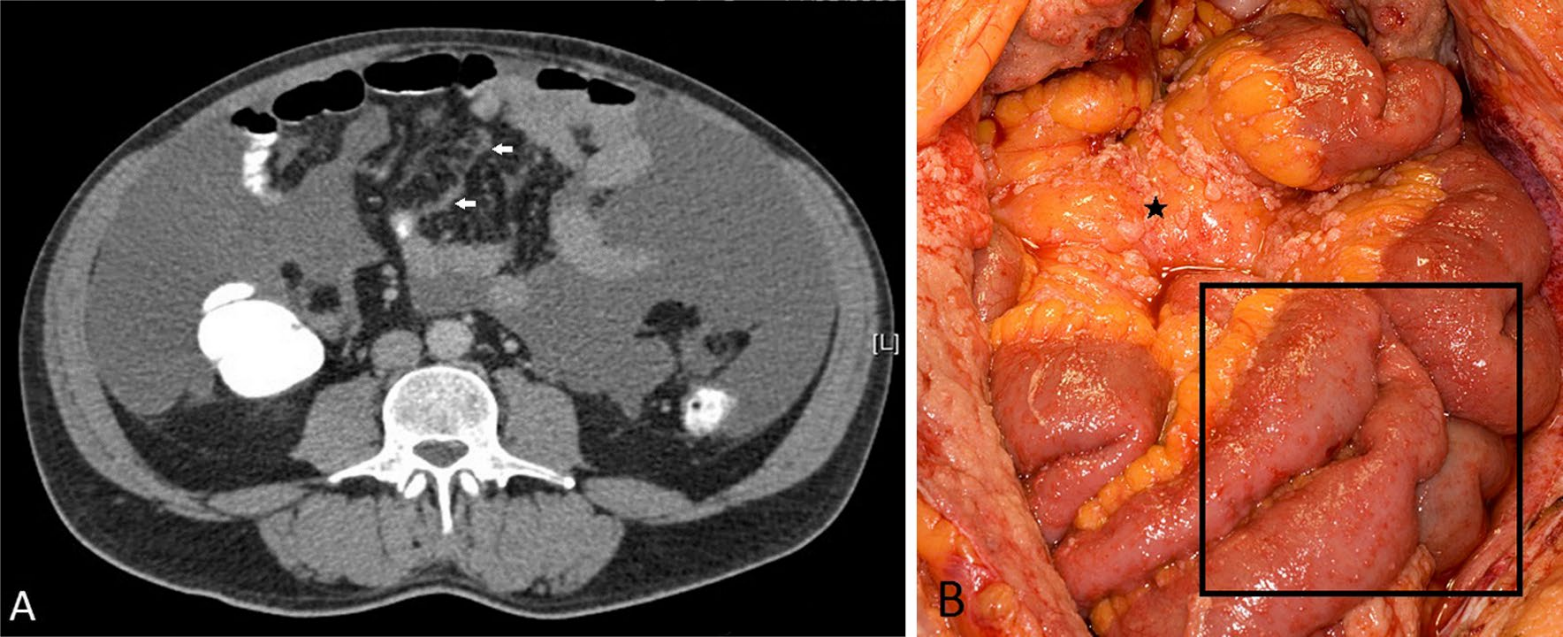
a, b Patient with epithelioid peritoneal mesothelioma. a CT shows ascites and subtle mesenteric nodularity (arrows). b Photograph from diagnostic laparoscopy showing diffuse mesenteric nodules (asterisk) and diffuse small bowel serosal nodules (black rectangle)
